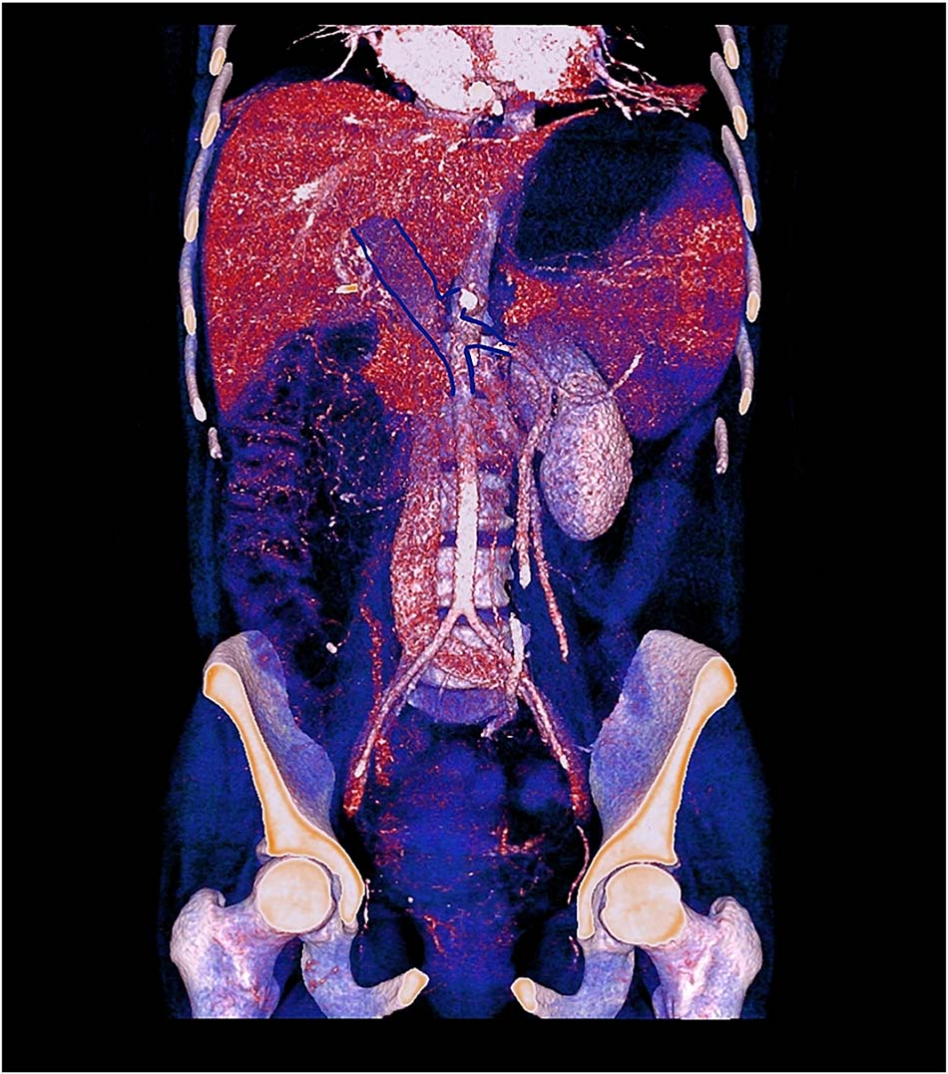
3D contrast-enhanced CT rendering reconstruction in venous phase showing thrombosis of the portal, splenic, and superior mesenteric veins with proximal dilatation and thickening of the jejunal wall.
